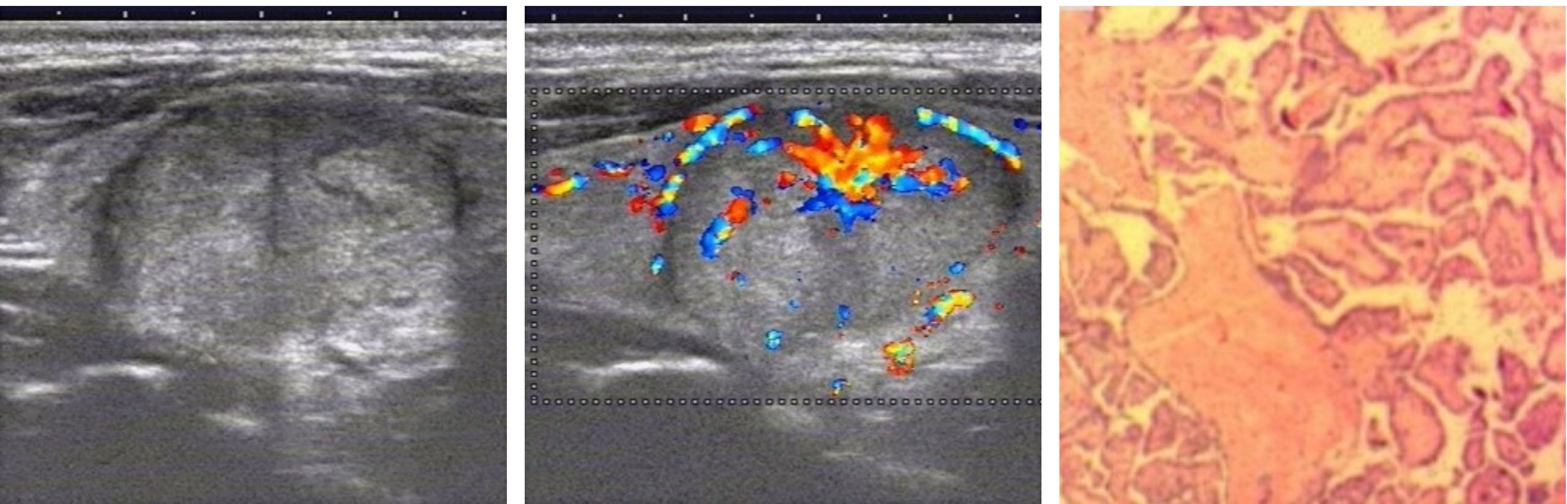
Grayscale ultrasonography shows a solid, isoechoic nodule in the right lobe of the thyroid with wider-than-tall and smooth margin and is considered to be a benign nodule on grayscale ultrasonography. Color Doppler ultrasonography showed the distribution of spoke wheel blood flow signals, which was determined to be papillary thyroid carcinoma. Postoperative pathology confirmed papillary thyroid carcinoma.
